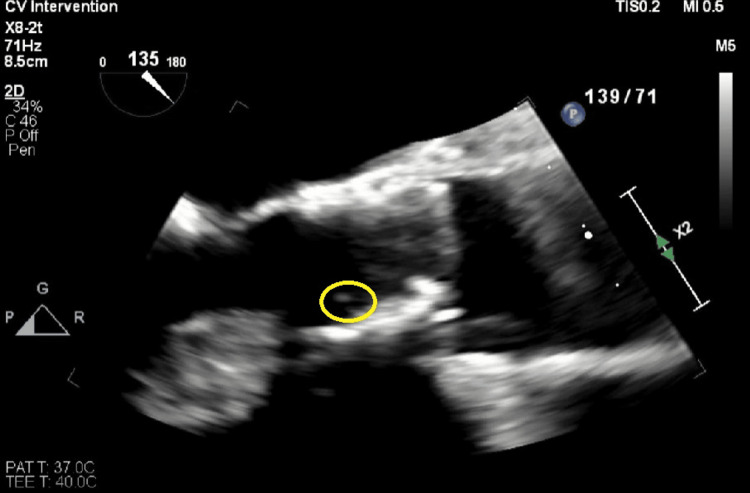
A transesophageal echocardiogram image showing an echo density measuring 1.1 x 0.2 cm (yellow circle) on the prosthetic aortic valve, consistent with a vegetation.
